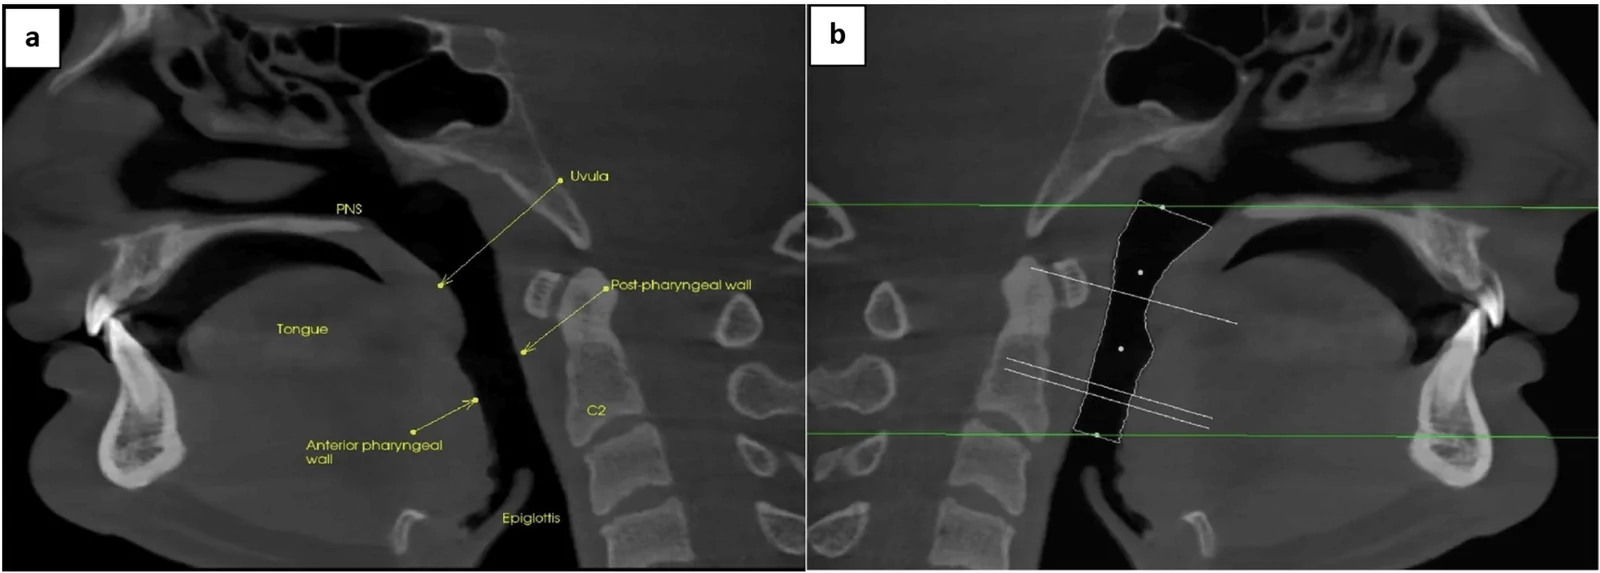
Anatomical basis for cone beam computed tomography (CBCT) airway measurements (a) Anatomical landmarks used to define the measured parameters, including the posterior nasal spine (PNS), soft palate, and posterior pharyngeal wall. (b) Superior and inferior boundaries of the airway region of interest, bounded superiorly by a line through the PNS parallel to the Frankfort horizontal plane, and inferiorly by a line through the antero-inferior point of the second cervical vertebra (C2).
